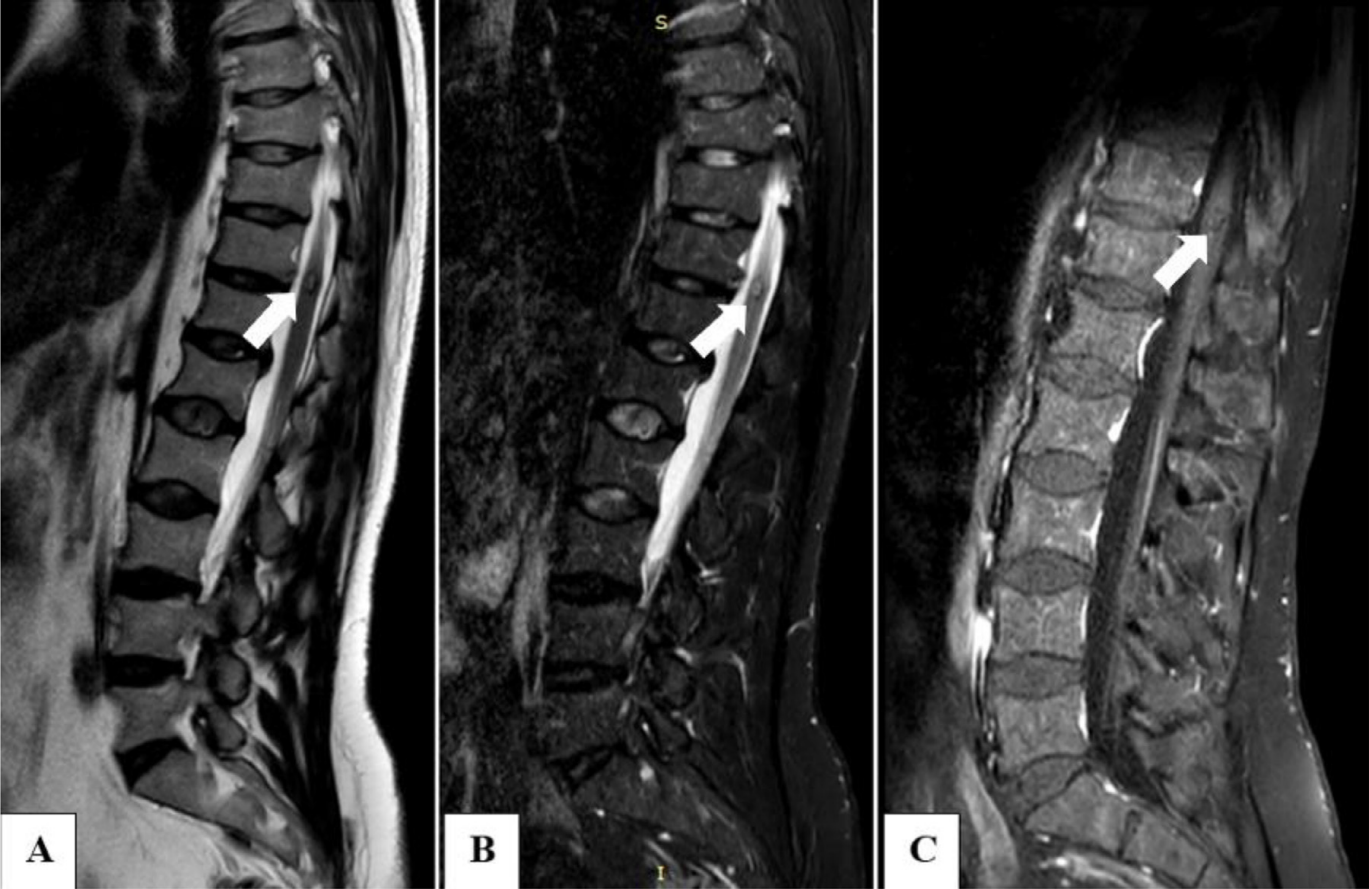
(A) Sagittal T2-weighted MR image showing a well-defined lobulated lesion with a center of mixed signal and a hypointense rim (arrow). (B) Sagittal T2-weighted MR image with fat suppression magnifying the typical ICM characteristics (arrow). (C) Sagittal T1-weighted-SPIR MR image with intravenous contrast material showing slight gadolinium enhancement of the lesion (arrow).
